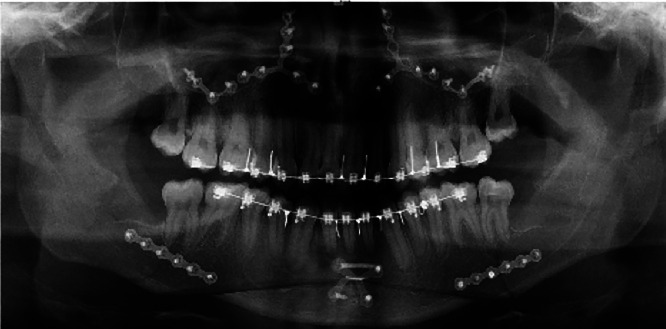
Postoperative panoramic radiograph. The arrows show an area of incomplete traces of the unwanted fracture.
